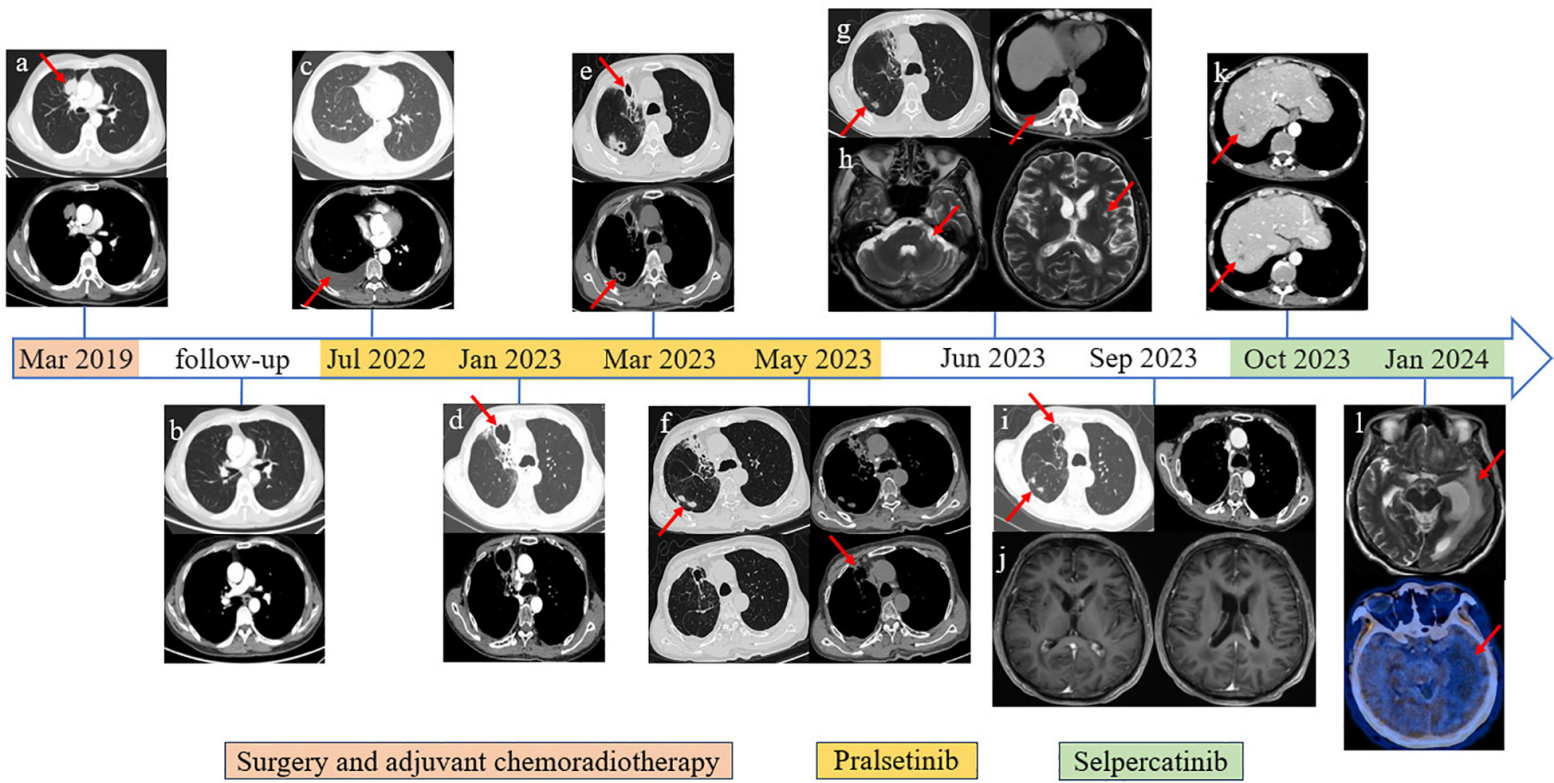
Imaging information throughout the disease course. (A) Primary lung tumor in the upper lobe of the right lung. (B) Follow-up chest CT scan image after surgery and adjuvant chemoradiotherapy. (C) Disease progression revealed by right pleural effusion cell smear. (D) Bronchial stenosis in the middle medial segment of the right lung, surrounding obstructive pneumonia and irregular cavity shadow, approximately 45 * 42 mm in size. (E) Cavity in the middle medial segment of the right lung was smaller than before, approximately 21 * 22 mm in size; newly added multiple irregular lesions with cavities. (F) The lesions on the middle medial segment of the right lung were unchanged. (G) The lesions were the same as above; recently presented right pleural effusion. (H) Abnormal enhancement in bilateral lateral ventricles and left cerebellar angle area. (I) The lesions on the middle medial segment of the right lung had shrunk. (J) The circularly enhancing lesion in bilateral lateral ventricles and left cerebellar angle area had shrunk. (K) Distant metastasis was discovered in the liver through the abdominal CT scan. (L) Cranial MRI revealed a new large edema on the left lateral ventricle compared with the previous examination, and further PET/CT (^18^F-FET) scan showed that there was no abnormal nuclide concentration in the edema area.
